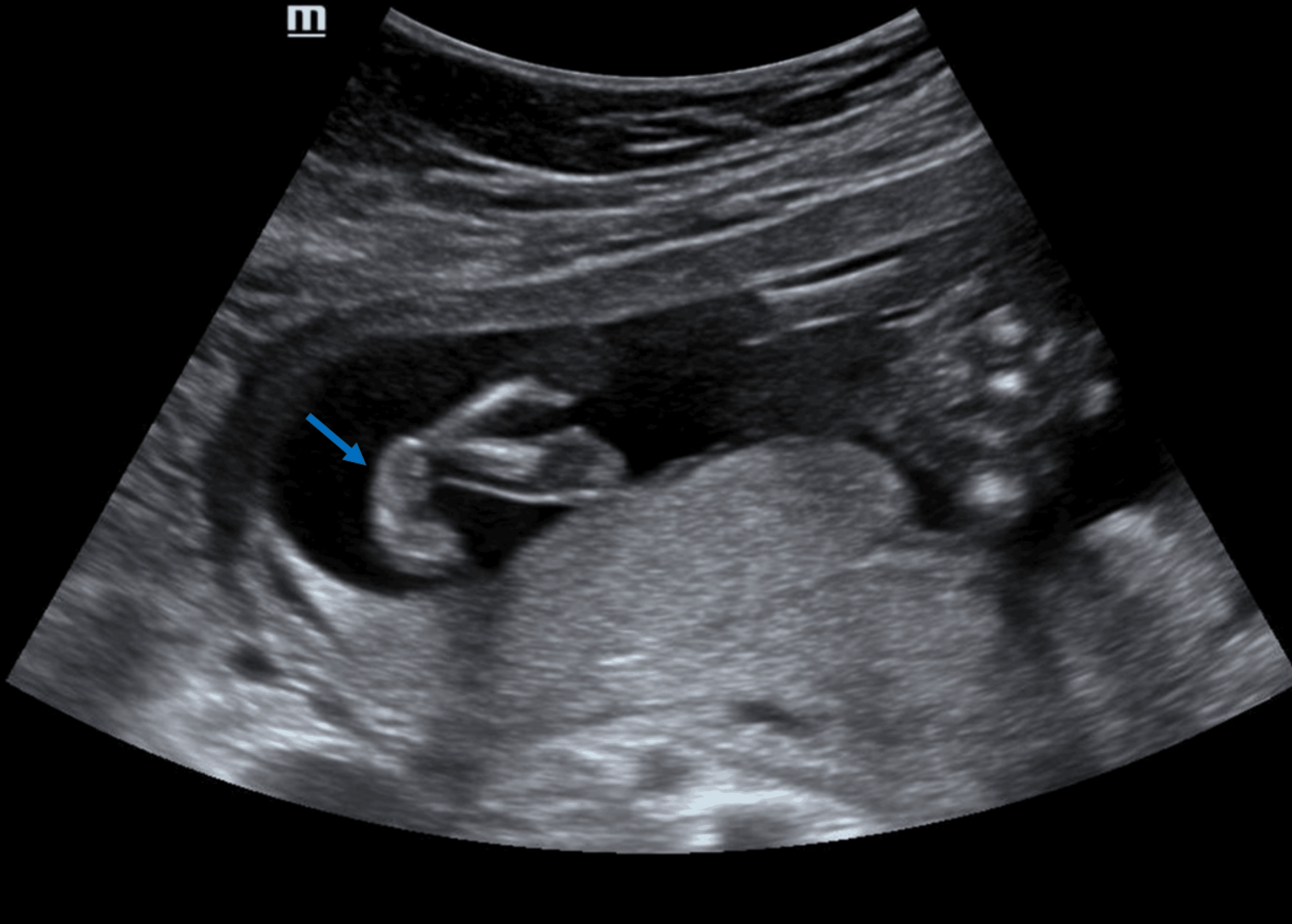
Ultrasound image of the fetal limb shows clubfoot (arrow).
